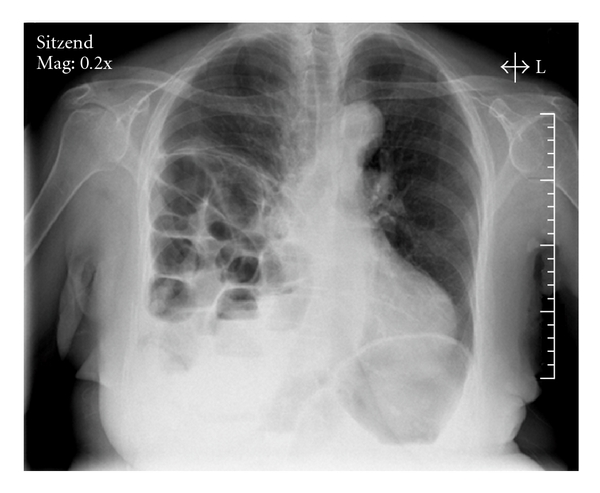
Chest radiograph shows bowel herniation into right hemithorax.
